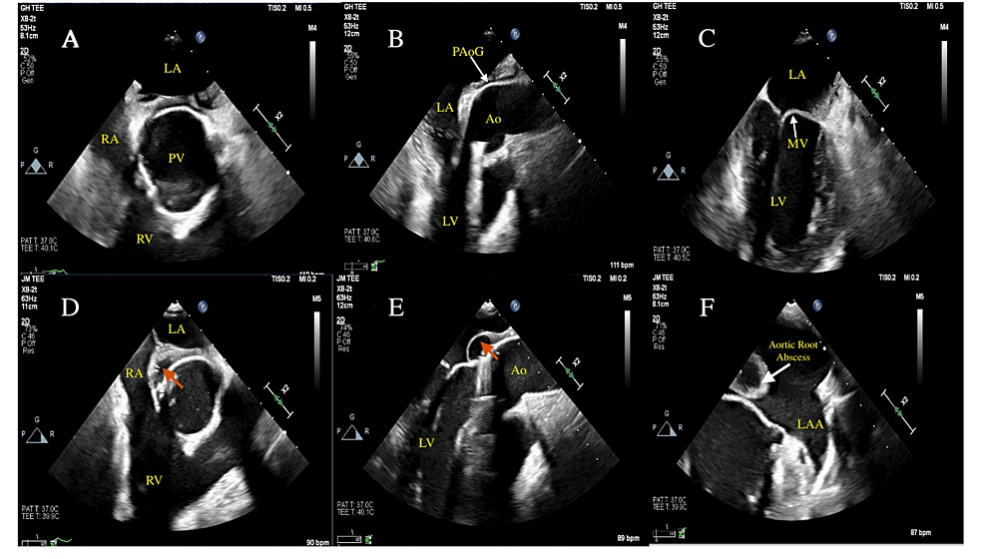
Invasion of the infection into the paravalvular structures (A-C) Transesophageal echocardiography images at initial diagnosis. (A) Short axis view across the prosthetic aortic valve (PV), right atrium (RA), right ventricle (RV). (B) Aorta (Ao) and prosthetic aortic graft (PAoG) can be seen in this view. (C) Mitral valve (MV), left atrium (LA), and left ventricle (LV) can be seen in this two-chamber view. (D-F) Transesophageal echocardiography after five weeks of intravenous antibiotics. Paravalvular abscess (red arrow) can be seen in (D) and (E). (F) Aortic root abscess can be seen in very close proximity to the MV, left atrial appendage (LAA) with no evidence of filling defects.
